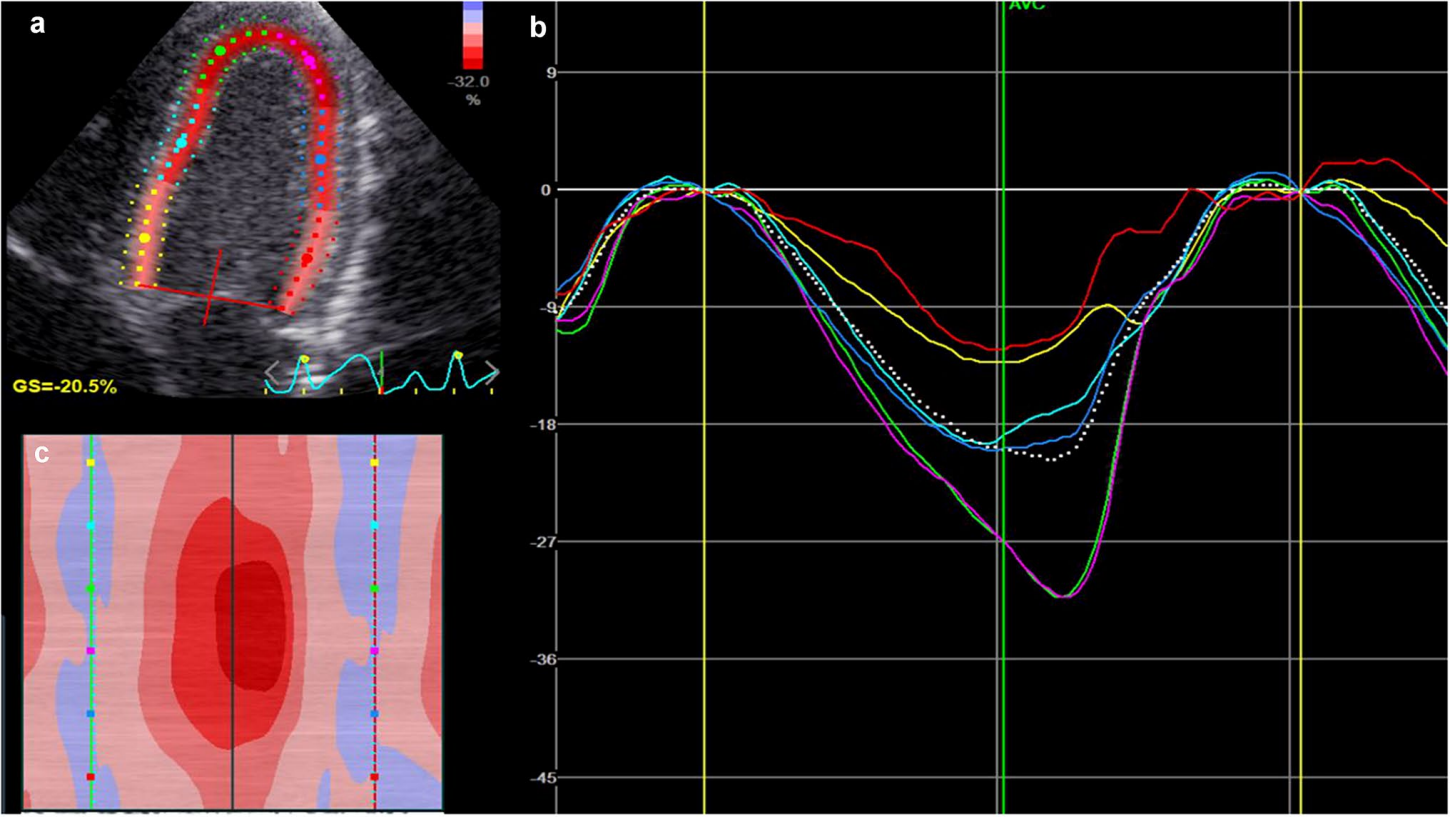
An example of a speckle-tracking echocardiography analysis. a The ultrasound image. b Six different curves with different colors, each corresponding to strain in a specific segment over time, in addition to the curve presenting average 4-chamber strain (broken curve). c Temporal changes in strain for each strain value, with high strain values represented as a darker red color
